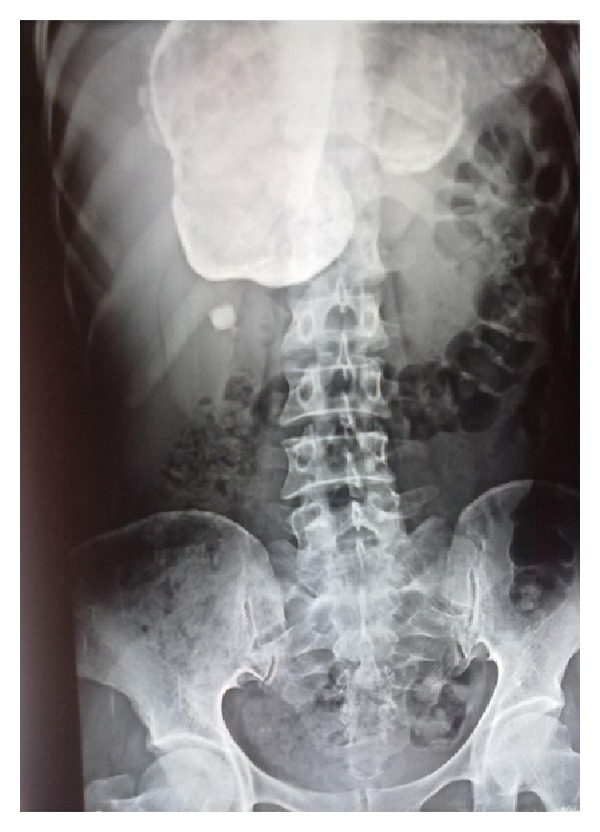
X-ray showing calcified hydatid cyst of liver.
